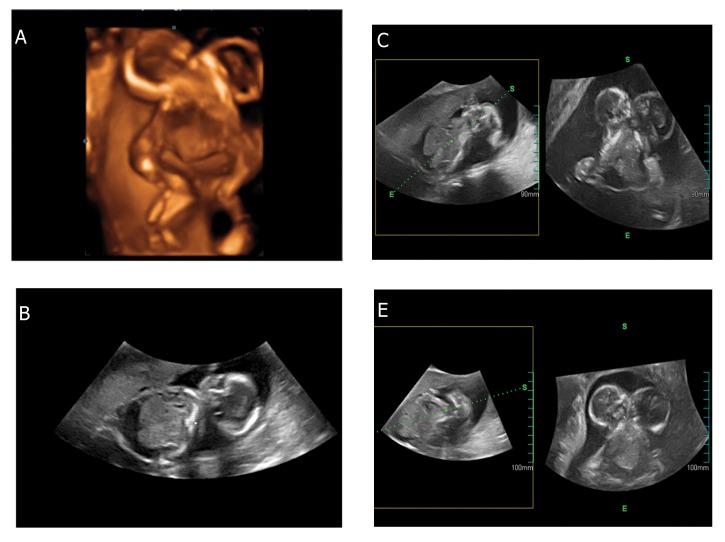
Ultrasound of conjoined twins (CT). These individuals
